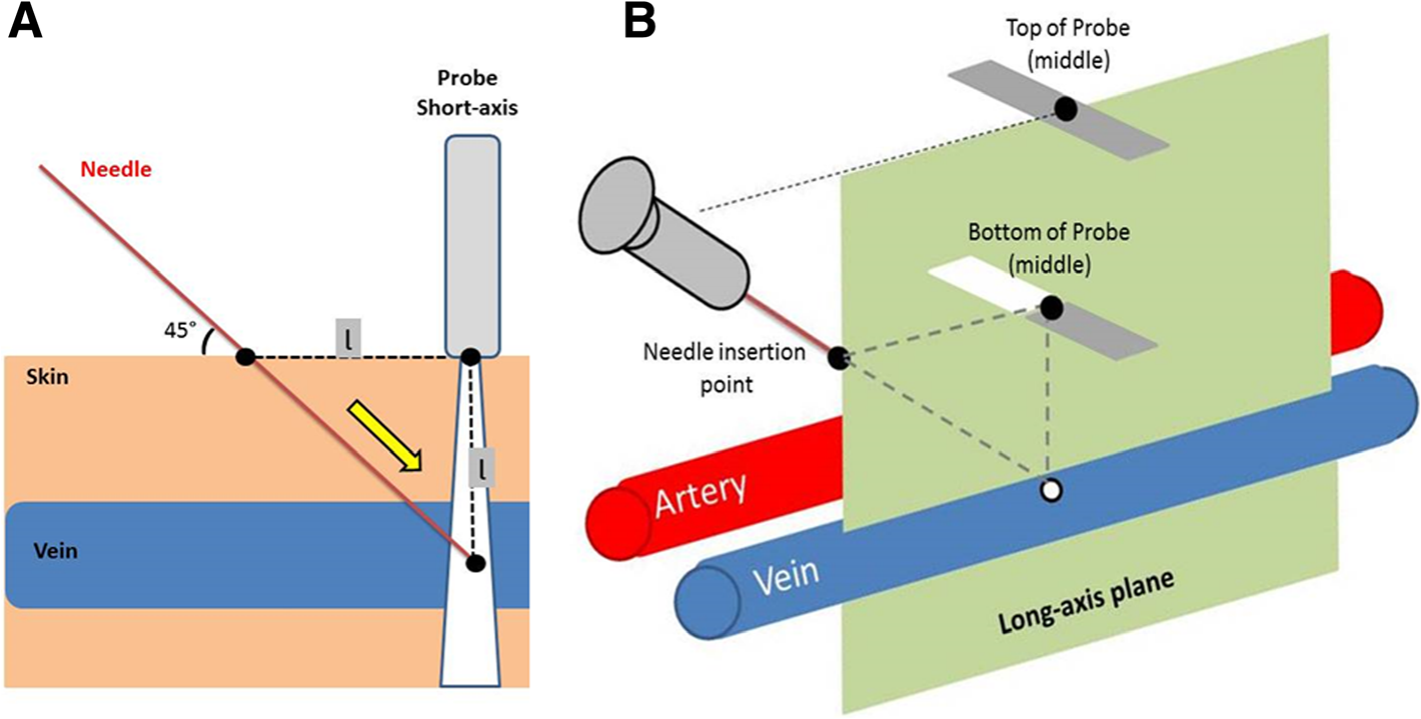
Global overview of ultrasound-guided vessel puncture and use of the isosceles right triangle. a Taking a distance between the probe and the needle insertion point equal to the depth of the vessel, a probe perpendicular to the skin, and an angle between the skin and the needle of 45°, the third angle will be automatically positioned into the vein. Three points build an isosceles right triangle: the needle insertion point, the middle of the probe on the skin interface, and the center of the vein under the probe. b The long-axis plane, which passes through the length of the vessel, corresponds to the view obtained in the in-plane technique with the vessel in the long-axis. Three points determine the long-axis plane: the needle insertion point, identified by inserting the needle in the skin just above the vessel, the vessel being viewed in the long axis; the middle of the probe on the skin, the vessel being visualized at the center of the ultrasound screen in the short axis; the middle of the probe’s top, the probe being perpendicular to the skin. The operator should attempt to maintain the syringe in the axis of the probe top (*dotted line*)
